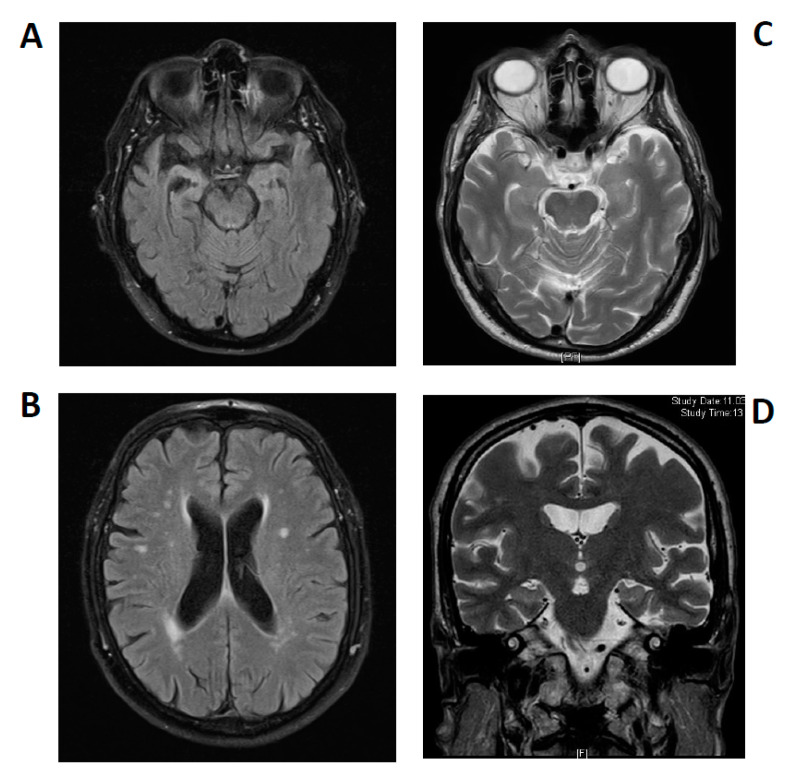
Brain magnetic resonance imaging. Patient 1: Asymmetrical atrophy of mesiotemporal structures in the right hemisphere and leukoencephalopathy (A,B): T2-weighted fluid-attenuated inversion recovery (FLAIR). Patient 2: Arachnoid cyst paramedial in posterior skull, but no further pathology (C,D) T2-weighted sequences).
